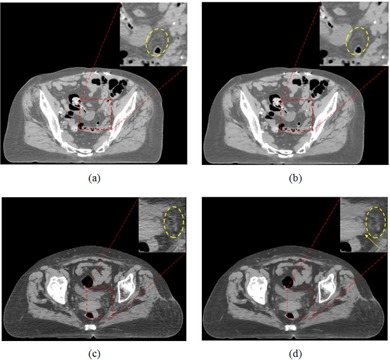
Assessment of anatomy conspicuity and realisticity for the CT images produced by the ${\text{iDose}}^{4}$ algorithm with (a) and (c) noise‐reduction‐level 1, and (b) and (d) noise reduction level 6.
